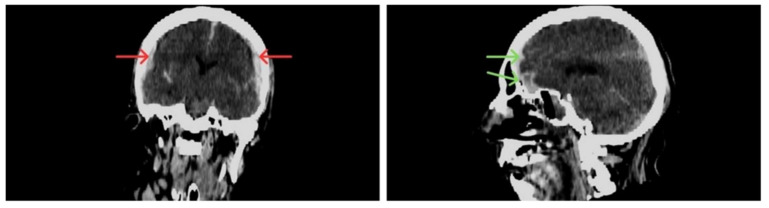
Post-impact CT scans of the subject’s head taken in the emergency department upon admission. The subdural hematoma is indicated with the red arrows and the frontal subarachnoid hemorrhage is marked with the green arrows.
